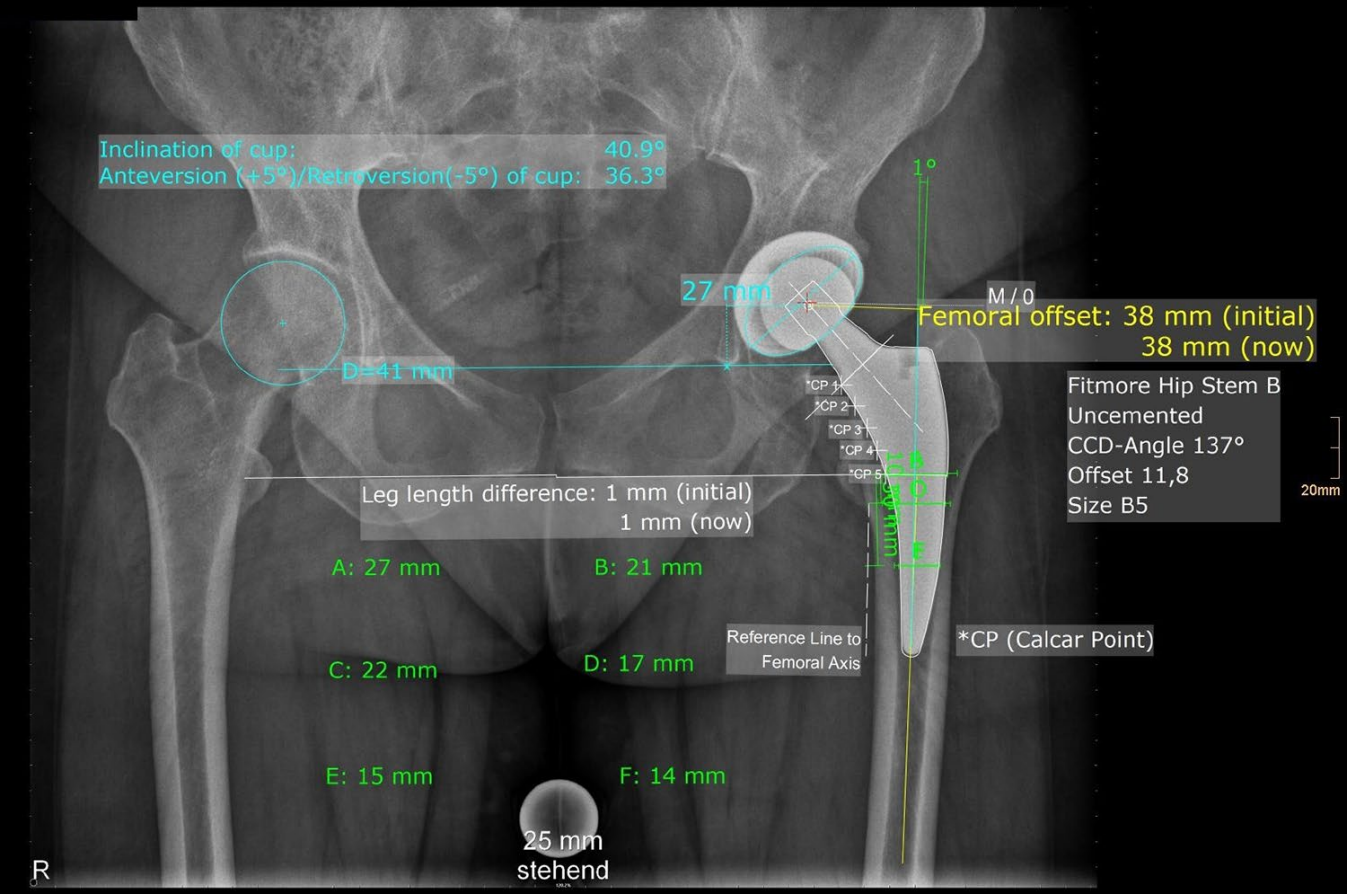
Postoperative X-ray with measurement with femoral offset (FO), acetabular offset (AO), leg length difference (LL), stem alignment, cup inclination and anteversion, Canal Fill Indices (CFI) I, II and III
